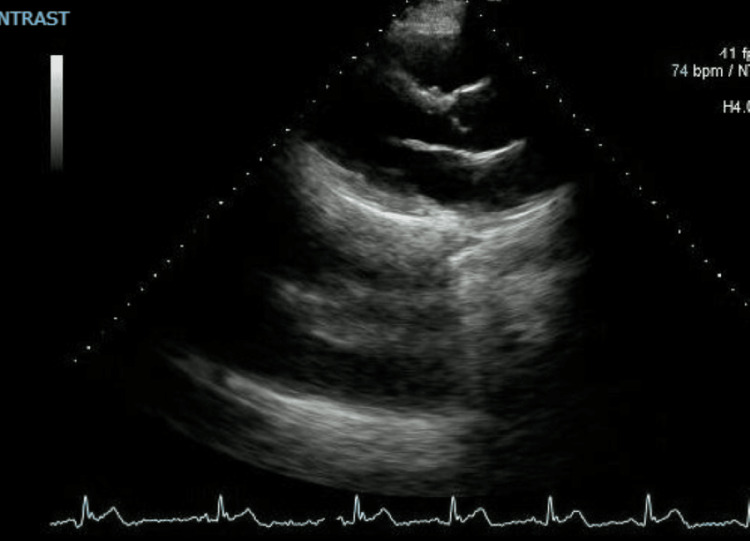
Transthoracic echocardiogram of the patient
